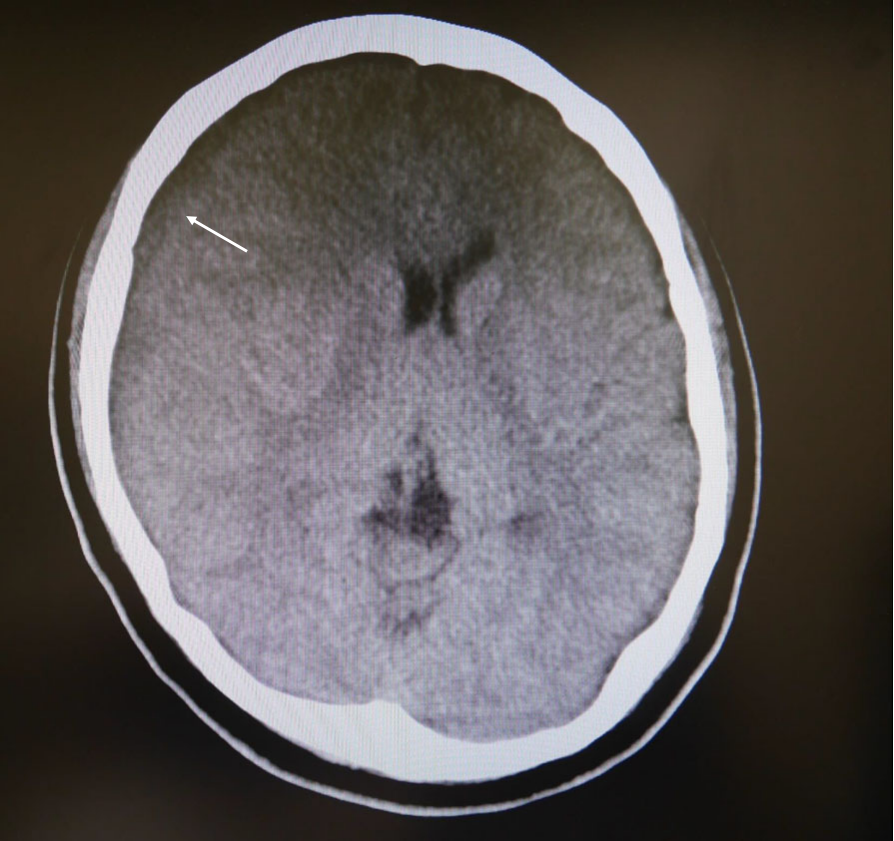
1 week after epidural blood patch was performed, computed tomogram shows resolution of the subdural hematoma (*arrow*)
